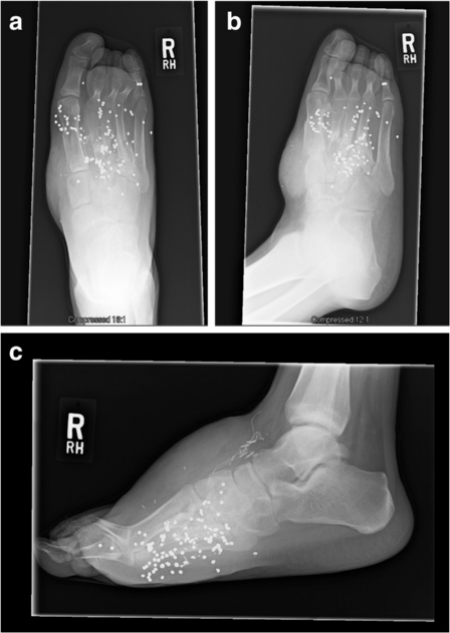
AP (a), oblique (b), and lateral (c) radiographs of the right foot 4.5 months post-op.
